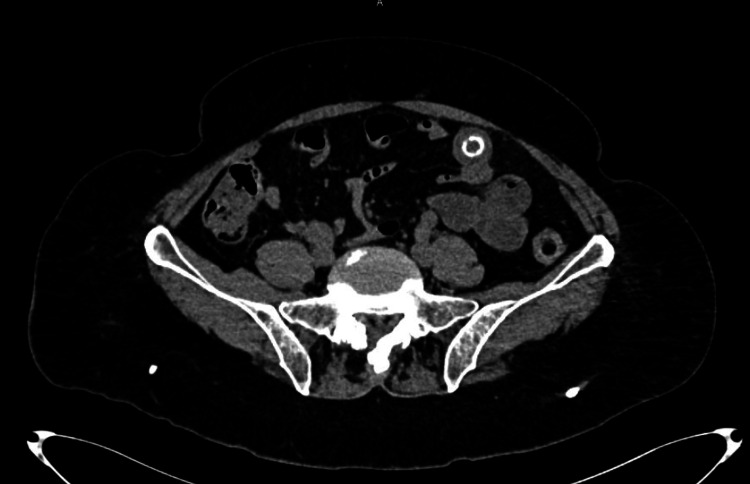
Axial View of Abdominal CT Scan A hyperdense image consistent with a gallstone measuring 13.2 mm × 22.1 mm was observed, with dilation of the small bowel loops. CT: computed tomography
